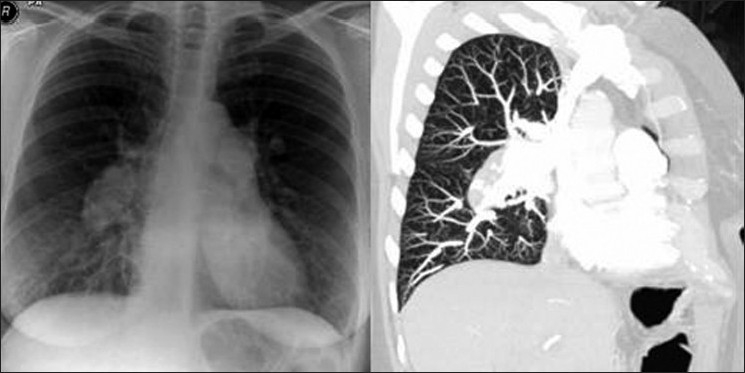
A CXR on a patient with PPH secondary to cirrhosis shows a dilated pulmonary conus and aneurismal dilatation of the right pulmonary artery. The image on the left is coronal reconstruction of contrast enhanced CT on the same patient, which depicts a probable re-canalized thrombus underlying the aneurismal dilatation of the right pulmonary artery
